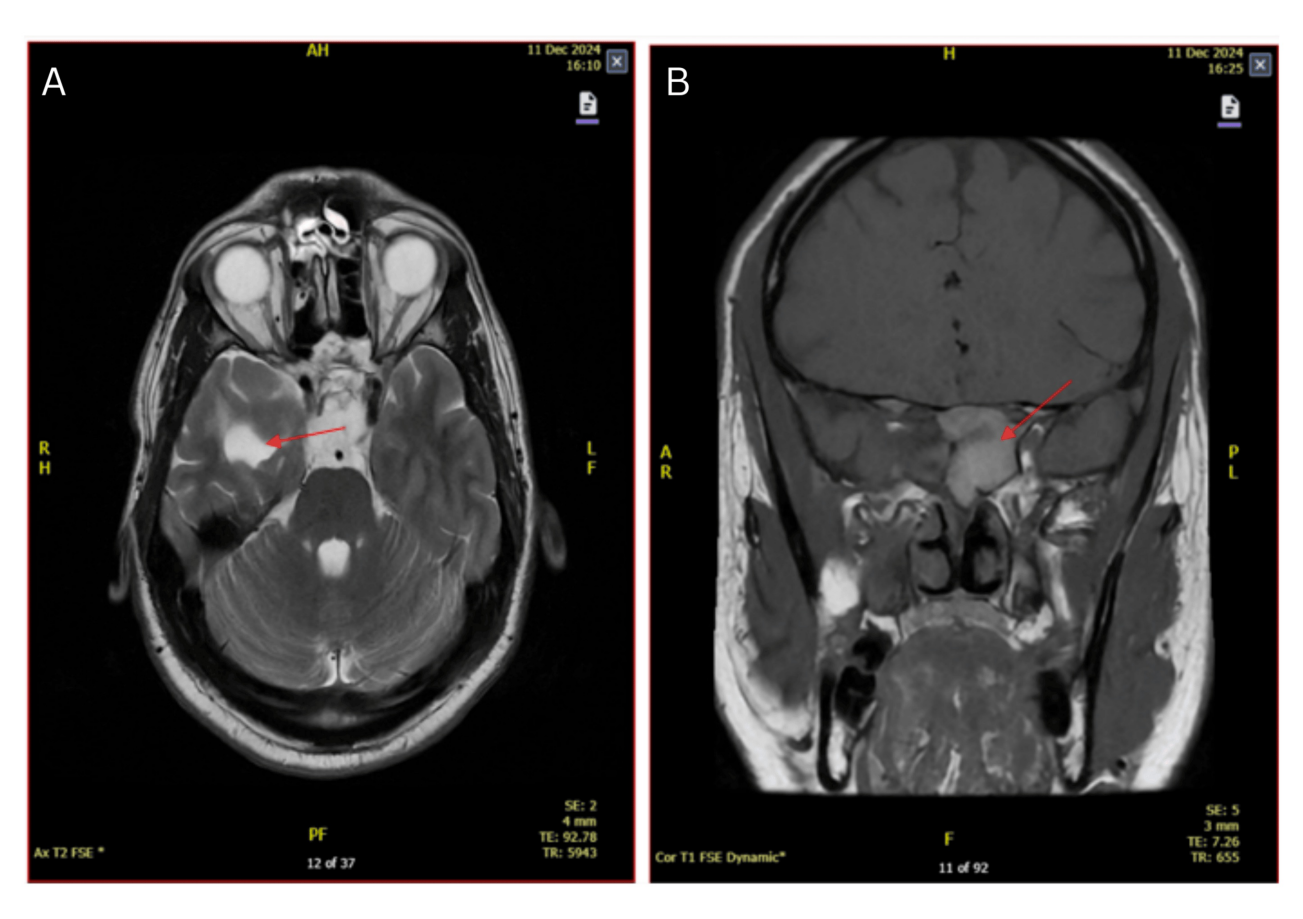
2024 MRI brain post-cabergoline treatment depicting partial reduction of pituitary tumour (shown with red arrows). A: Transverse view; and B: coronal view.
